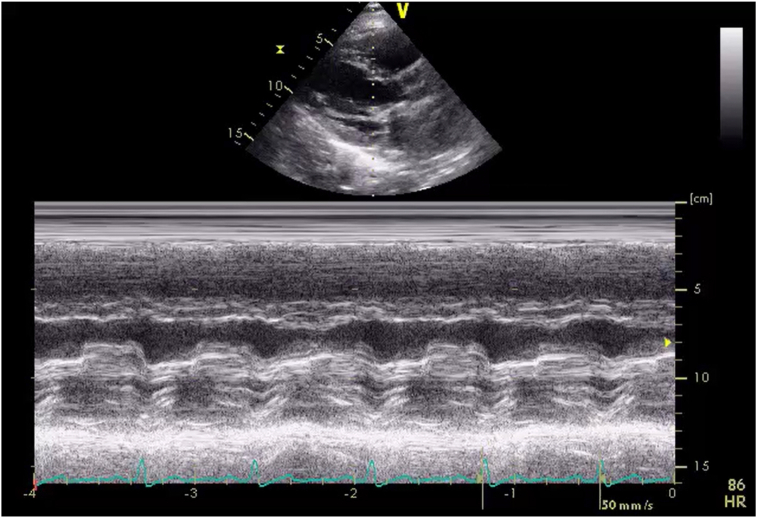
M-mode TTE, parasternal long-axis view, demonstrates a markedly reduced diastolic excursion of the mitral leaflets, reduced E-F slope consistent with MS, and a mass obscuring the mitral orifice.
